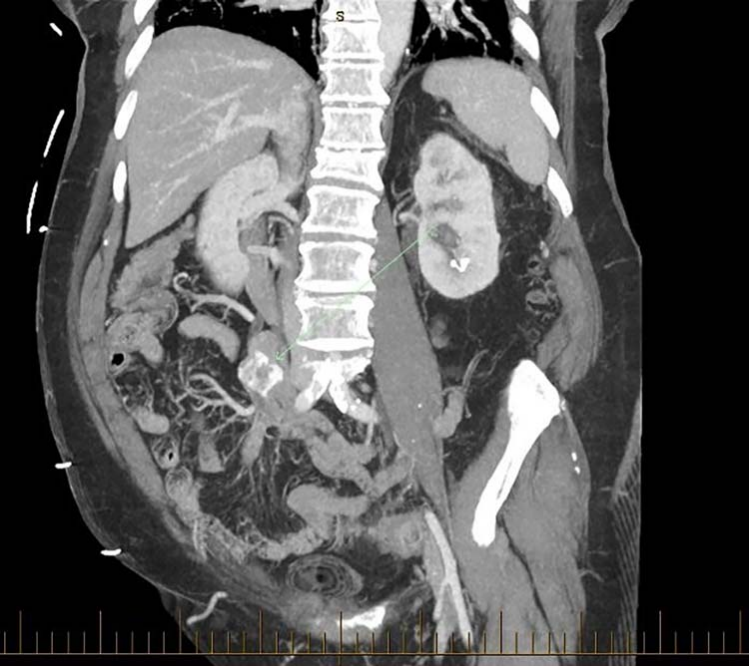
Coronal CT BC embedded in the distal jejunum.
